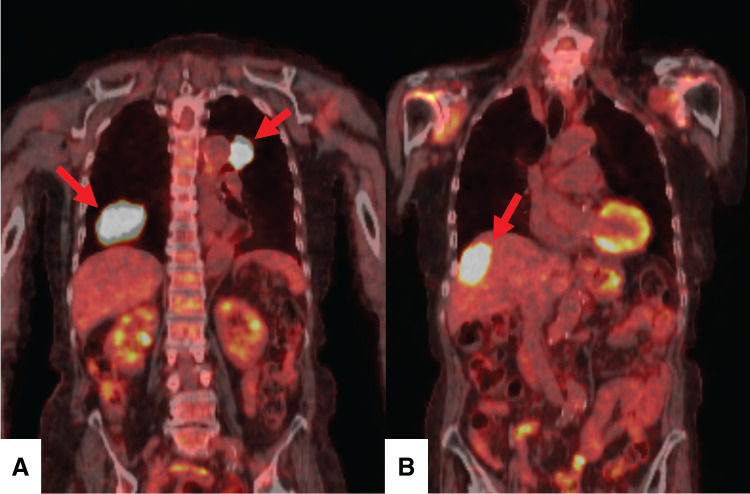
FDG-PET/CT in year 2024 showed the accumulation of FDG in (A) the right S^6^ and left S^1+2^ lung tumors (arrows) and (B) the liver tumor (arrow), but no accumulation in other areas. The patient was diagnosed with stage IVB lung cancer with multiple metastases in multiple organs. FDG-PET, ^18^F-fluorodeoxyglucose positron emission tomography
